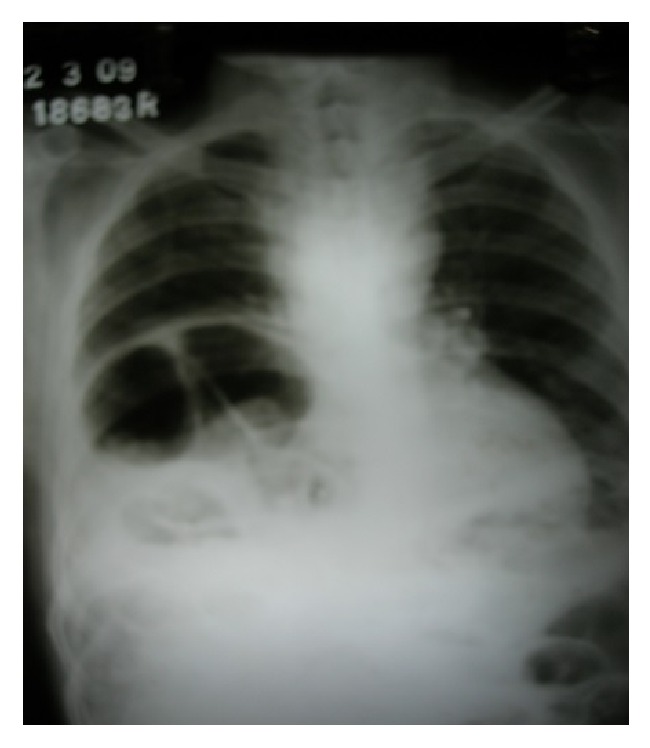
Chest X-ray showing an elevated right hemidiaphragm.
